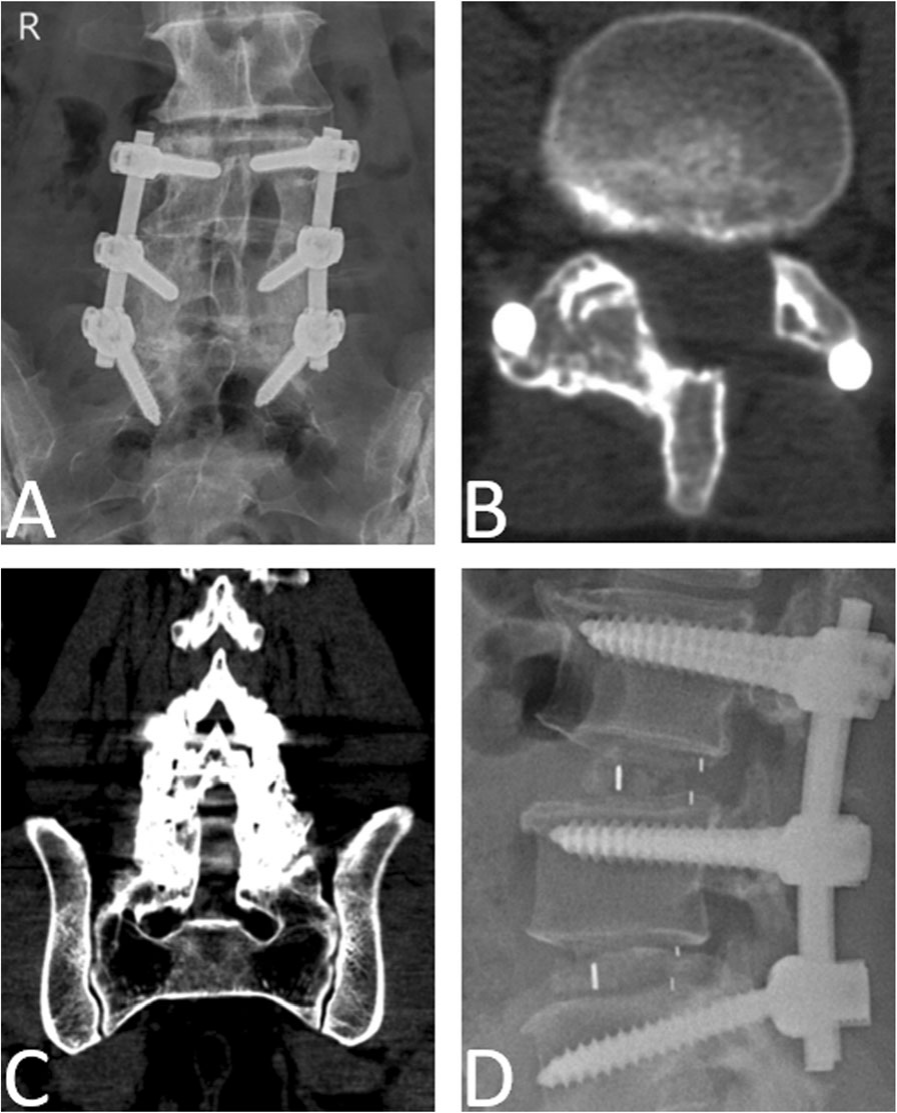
Fusion standard. a Lumbar radiography and b axial CT showed that a continuous bony bridge had formed between the right L4–S1 laminae and right L4/5, and the L5/S1 facet joint space was replaced by an osseous connection. c Anteroposterior CT showed that there were continuous bony bridges between the L4 and S1 transverse processes at the two sides (Grade A). d Lumbar radiography showed that there were continuous bony bridges in the L3/4 and L4/5 intravertebral spaces
